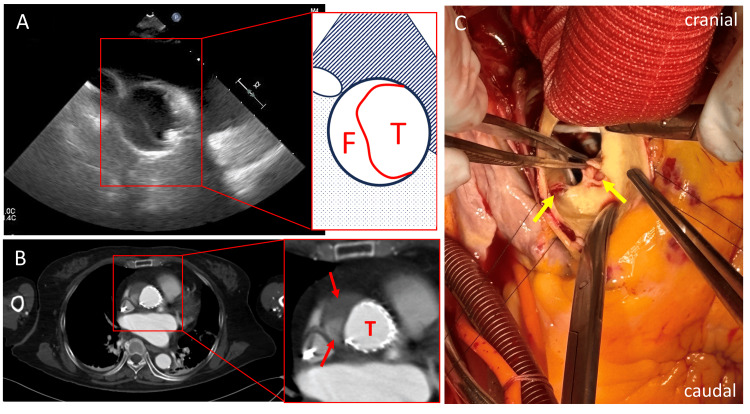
Aortic dissection and intimal tears (A) Transesophageal echocardiography and (B) Contrast-enhanced computed tomography revealing Stanford type A acute aortic dissection. T: true lumen; F: false lumen; red curve indicates mobile intimal flap; upper red arrow indicates a hematoma within the false lumen; lower red arrow indicates contrast enhancement within the false lumen. (C) Intimal tears are in the sinus of the right coronary cusp (right arrow) and non-coronary cusp (left arrow).
